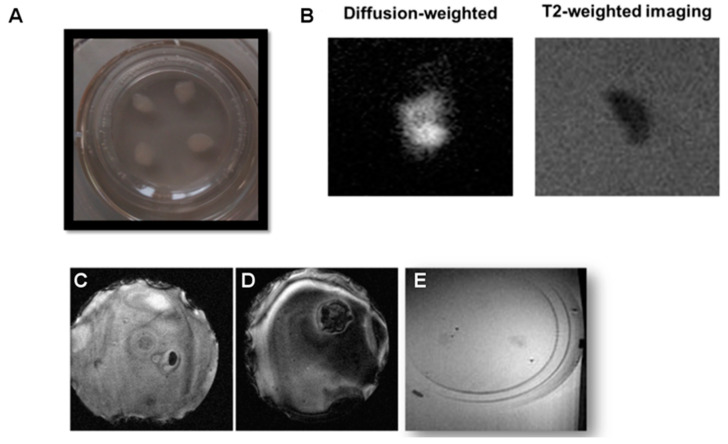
MR imaging of thin organotypic slices. (A) A photograph of organotypic slices in culture. (B) Diffusion-weighted vs. T2-weighted imaging of an organotypic slice. (C–E) Different imaging artifacts observed when imaging thin tissue slices ((C) air bubble; (D) misalignment of the supporting membrane with imaging plane; (E) loss of CNR due to partial volume effects).
